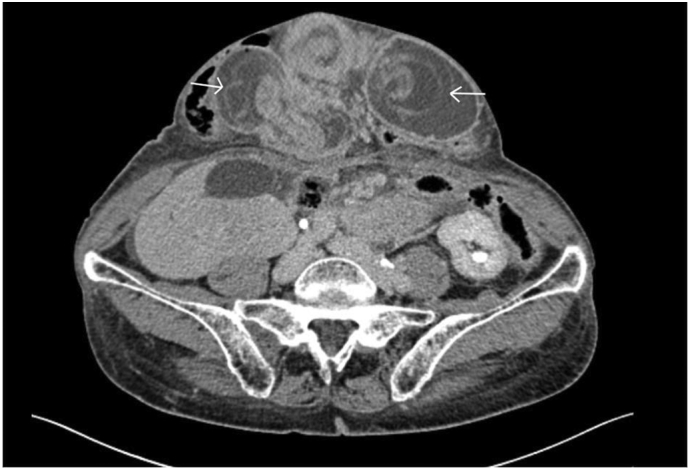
CT Images. Arrows indicate intussusception or “target sign”.
